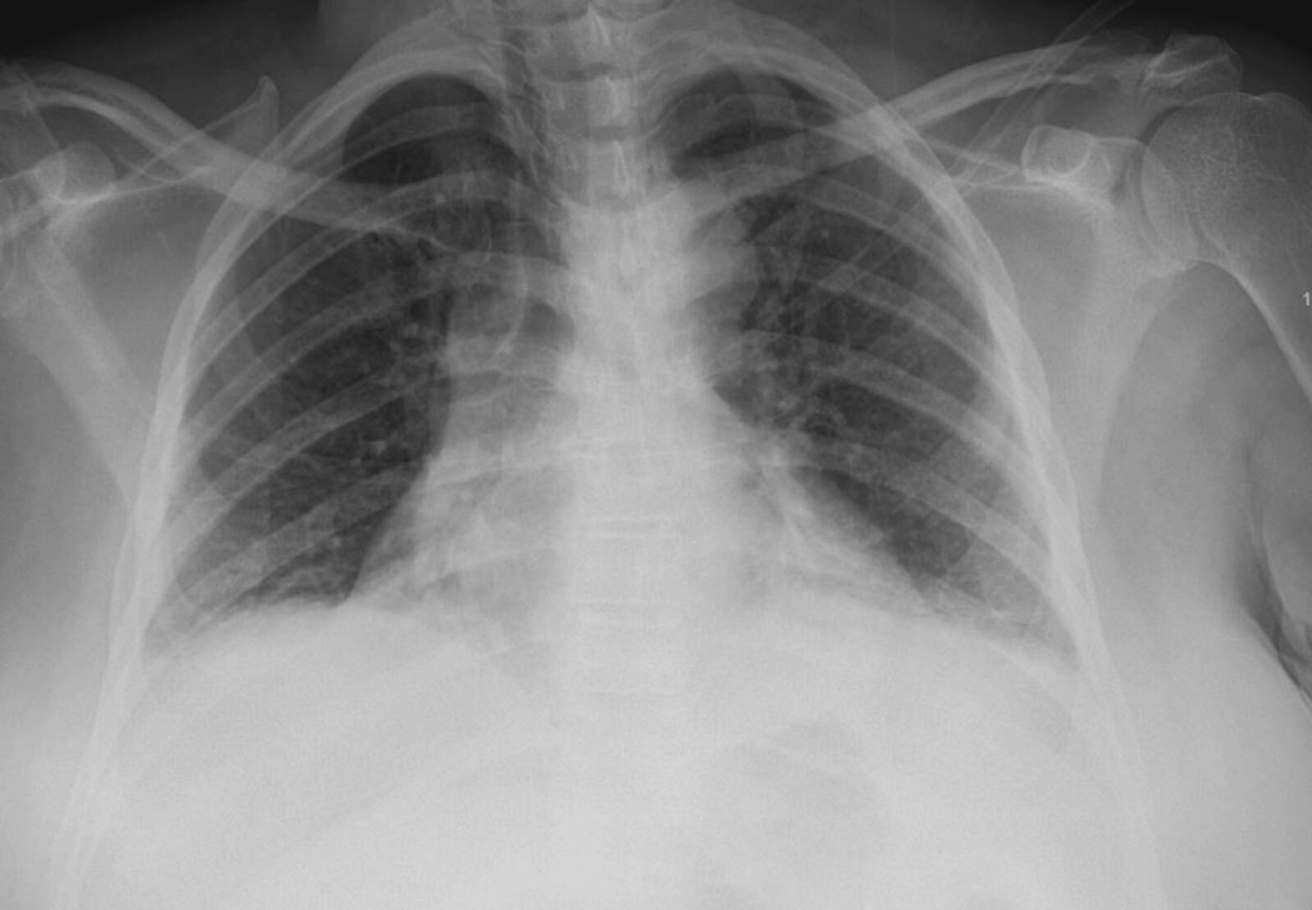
Portable chest X-ray showing atelectatic changes
